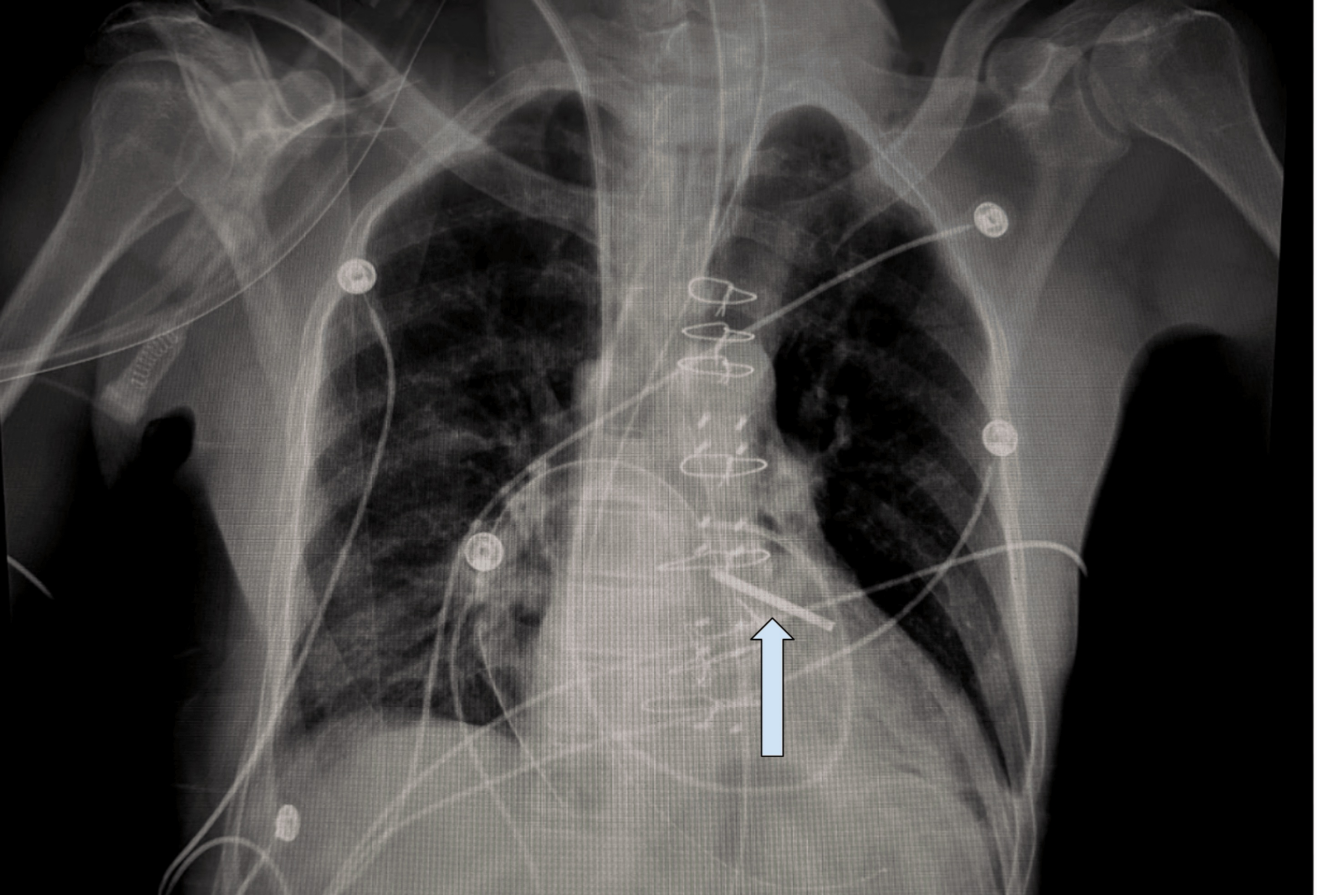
Postoperative chest X-ray depicting valve replacement and left atrial appendage clip (arrow).
